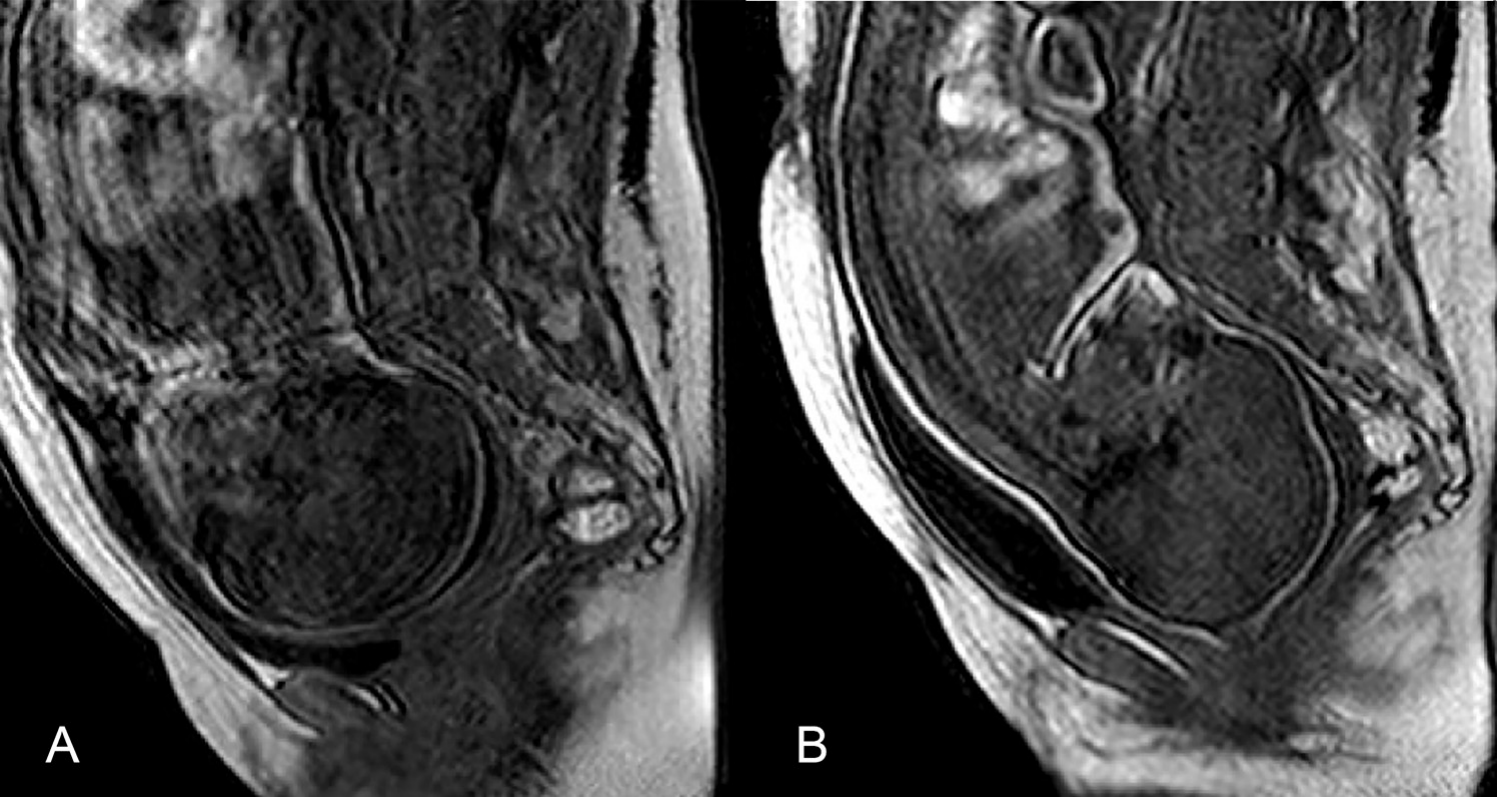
Median sagittal plane MRI before and during labor in patient 5. (a) No fetal head molding was observed before labor. The maternal coccyx was concave anteriorly, and the perineal raphe horizontal.; (b) The fetal head is shown in the middle pelvic brim during the second stage of labor. The fetal presentation has turned to occipito-pubic, the coccyx has tipped backward, and the perineal raphe has assumed a vertical position. The fetal head has typical “sugarloaf molding”. This head molding was not observed after birth.
